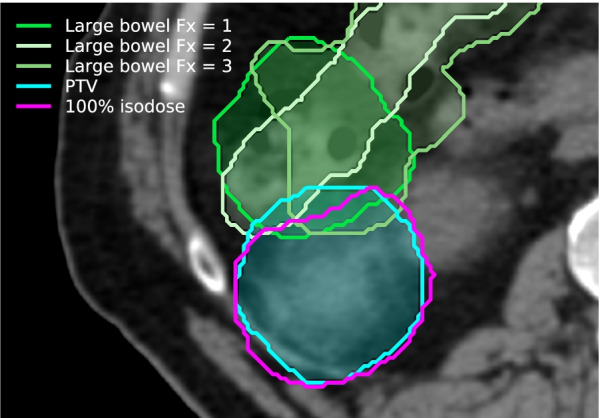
Large bowel (green) position as determined from PRE CBCT in fraction 1/2/3 of a patient that had bowel stricture surgery post SABR treatment. The PTV (cyan) and the 100% isodose line (magenta) are shown
